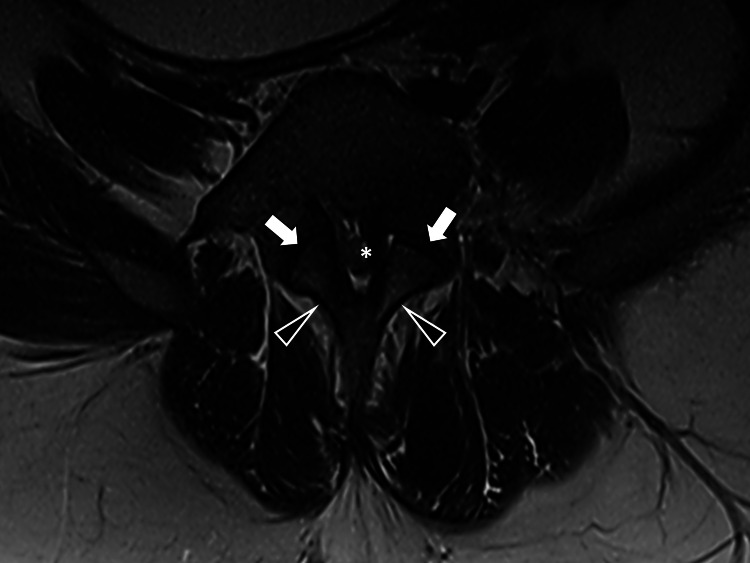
Magnetic resonance imaging for the spine in the axial plane Magnetic resonance imaging (T2-weighted axial view) at the level of L5 shows short thickened pedicles (arrows), extremely decreased transverse diameter of the spinal canal (asterisk) and thickened laminae (arrowheads).
